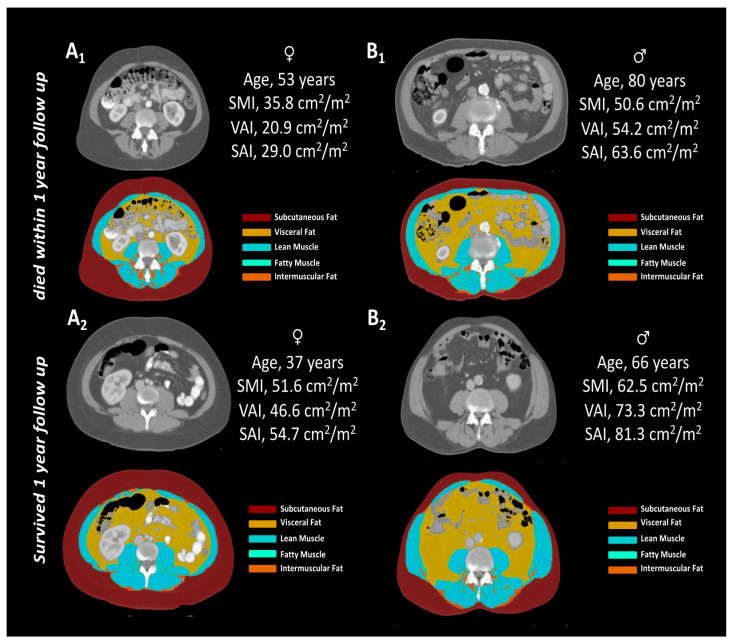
Exemplary patients from the study population. Female (A1,A2) and male (B1,B2) patients who passed away (A1,B1) or were alive (A2,B2) 1 year after initial staging CT for treatment initiation of immune checkpoint inhibitor therapy alongside obtained body composition metrics (SMI, VAI, SAI). Abbreviations: SMI, skeletal muscle index; VAI, visceral adipose tissue index; SAI, subcutaneous adipose tissue index.
